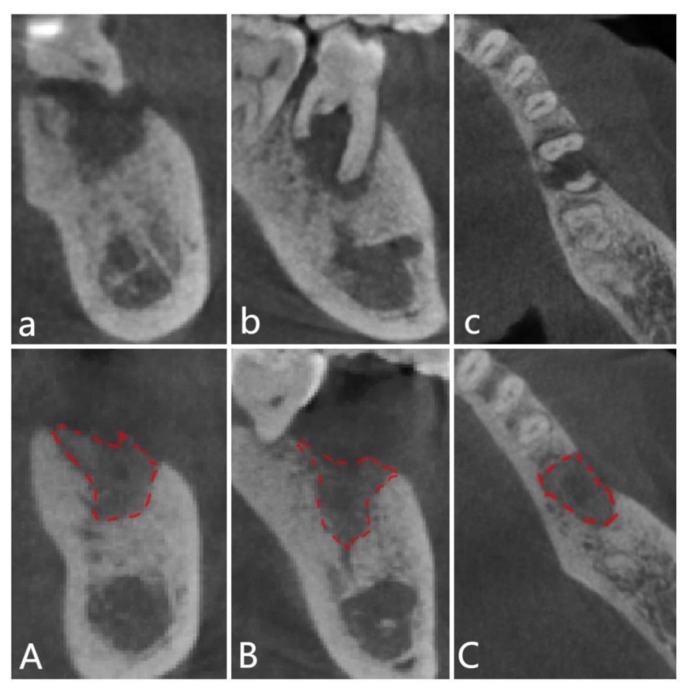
CBCT images of the (a,A) coronal; (b,B) sagittal; and (c,C) cross-sectional sections of the alveolar bone taken 6 months post-surgery. Red lines: Increased of the grafted site could be observed.
